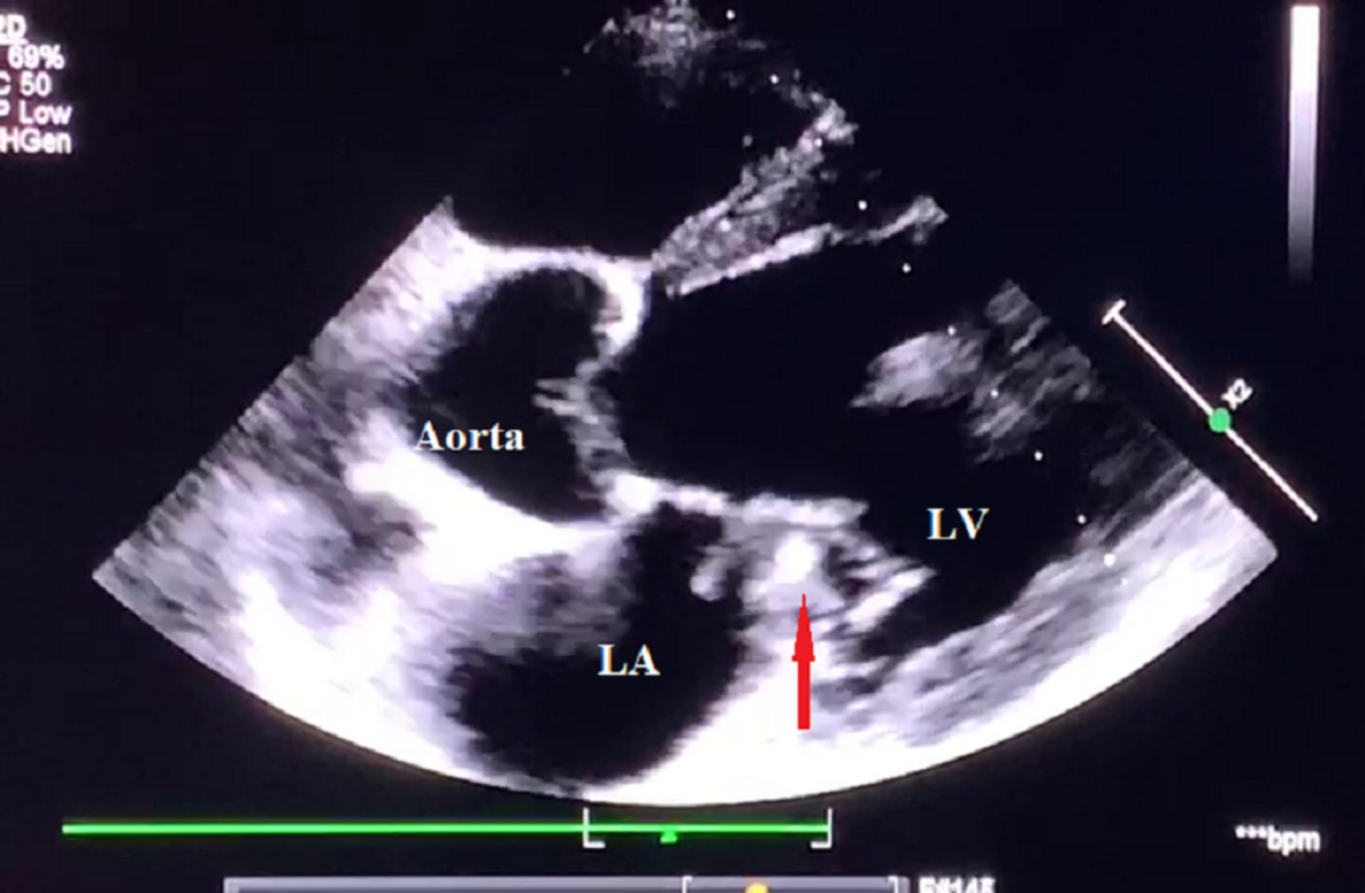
Trans-esophageal echocardiography Red arrow shows 7 x 12 mm vegetation attached to the anterior mitral leaflet.
